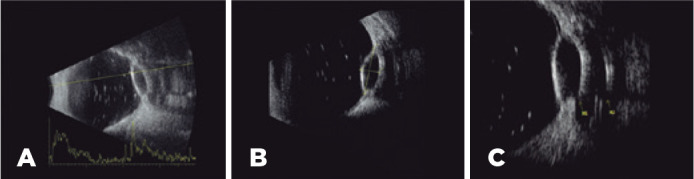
Ultrasonography showing residual emulsified silicon oil in the vitreous
